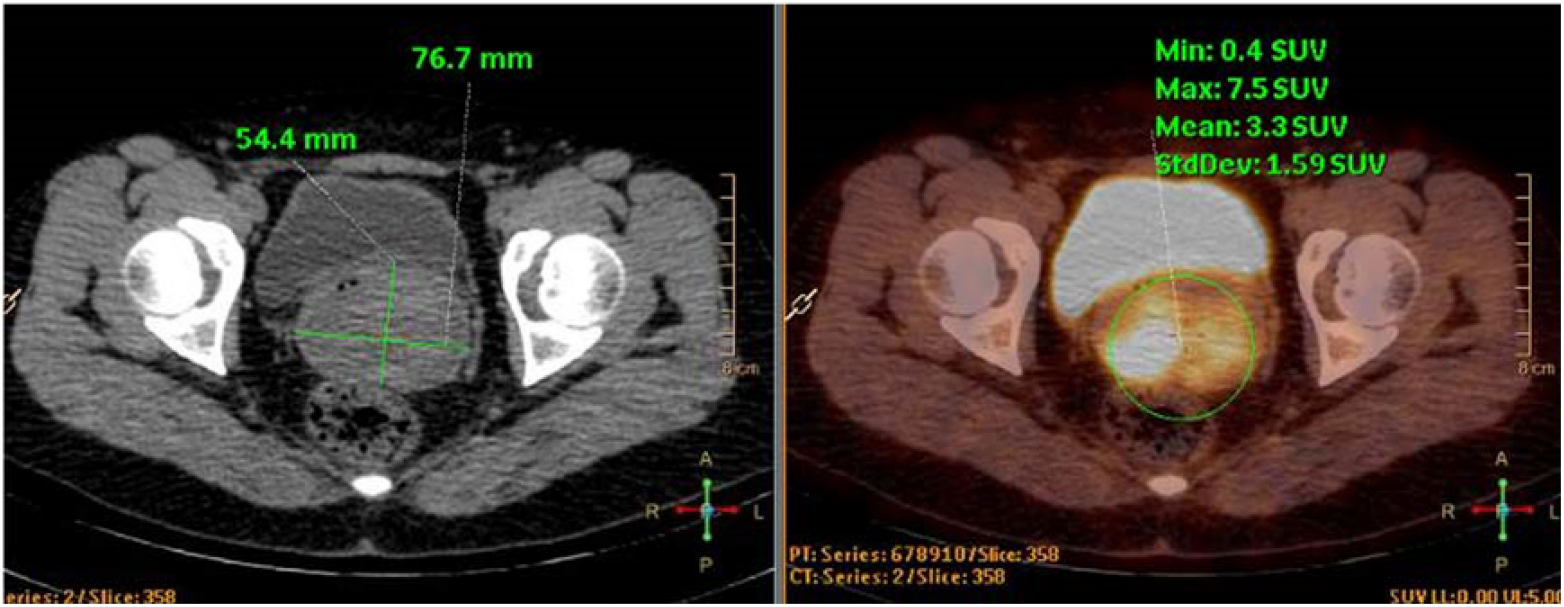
PET-CT images. The cervical lesion showed increased metabolic activity, consistent with features of a malignant tumor.
